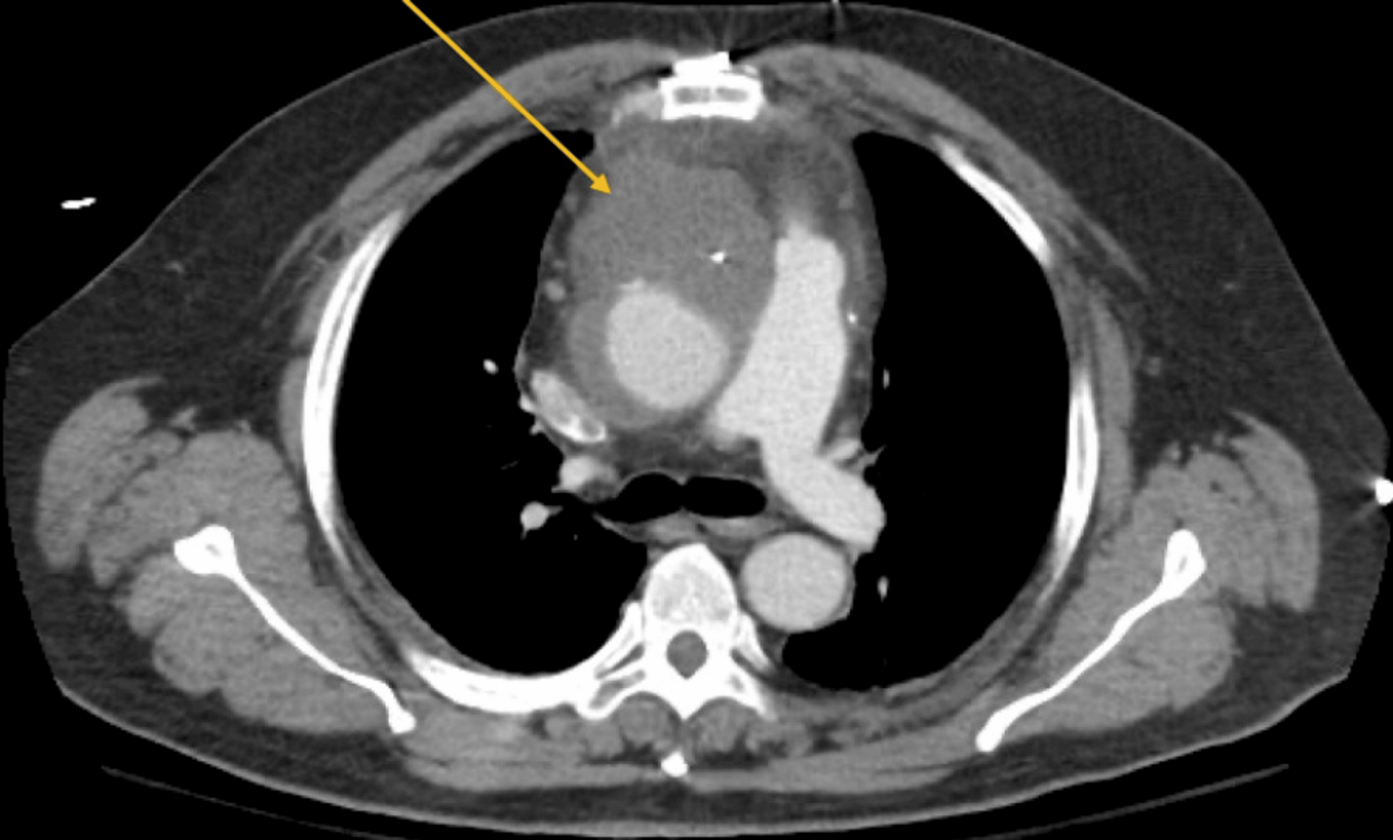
Initial CT imaging of the mediastinal mass.
